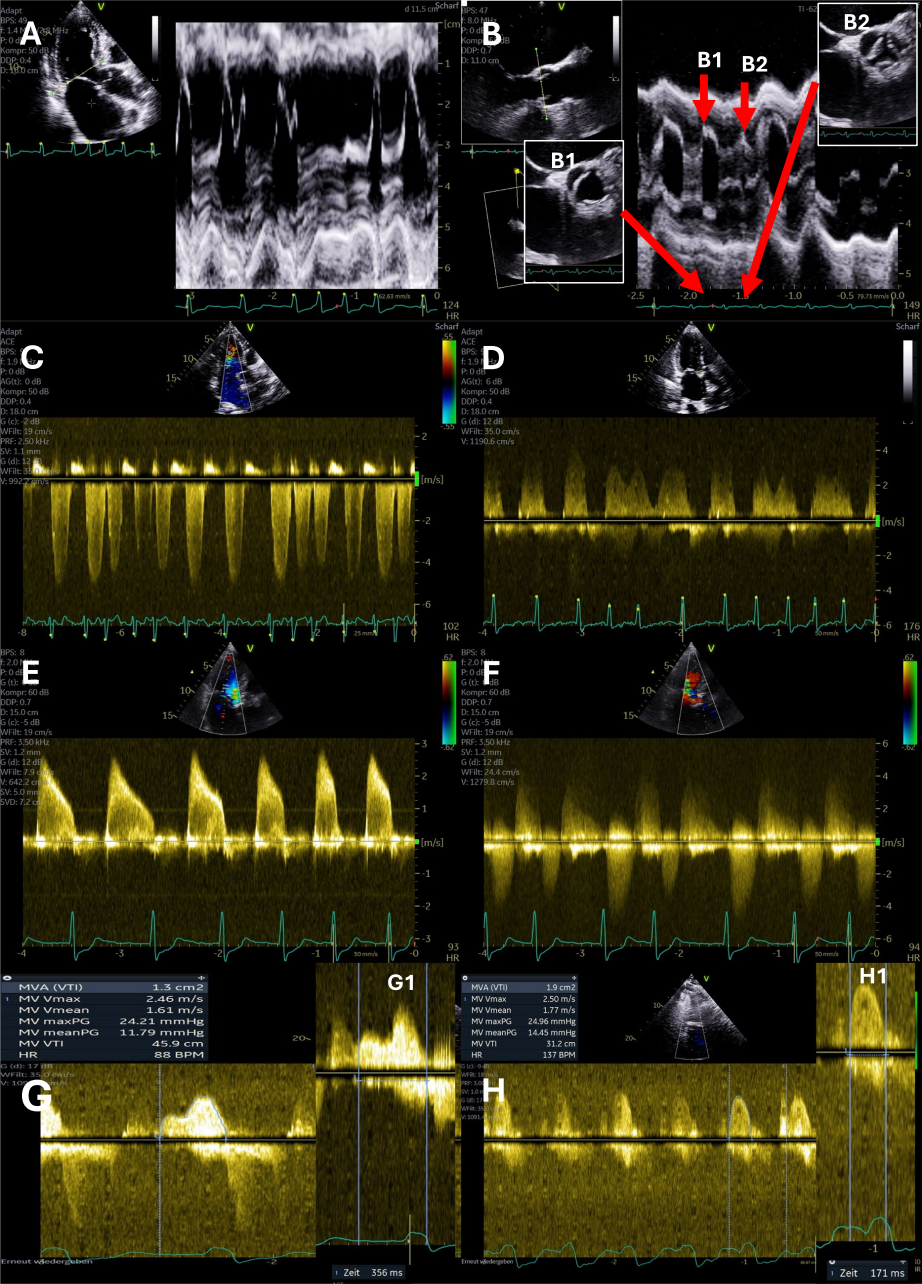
Effects of valvular heart diseases on hemodynamics in patients with atrial fibrillation (AF). A Anatomical M-Mode through the mitral valve (MV) separation during tachyarrhythmia—reduced valve separation is documented with shortening of RR-intervals; B biplane TTE (transthoracic echocardiography) views and anatomical M-Mode through the aortic valve separation area during AF—reduced valve separation is documented with shortening of RR-intervals—in addition, differences of maximum aortic valve (AV) opening in short axis views at representative RR-cycles (B1, B2); C continuous wave (cw) Doppler spectrum of the MV in a patient with mitral regurgitation during AF—reduction of MV inflow and increase of regurgitant flow is documented with increasing heart rate; D cw Doppler spectrum of the AV in a patient with aortic regurgitation during AF – increase of AV regurgitant flow is documented with increasing heart rate; E cw Doppler spectrum of the MV in a patient with mitral stenosis (MS) documenting the impact of increasing heart rate on transmitral flow; F cw Doppler spectrum of the AV in a patient with aortic stenosis documenting the impact of increasing heart rate on transaortic flow; G cw Doppler spectrum of the MV in a patient with MS (transmitral velocities and gradients are shown as well as diastolic duration in G1); H cw Doppler spectrum of the MV in the same patient with MS after switching to tachyarrhythmia (transmitral velocities and gradients are shown as well as diastolic duration in G2 documenting the impact of AF on transmitral inflow and transmitral gradient)
